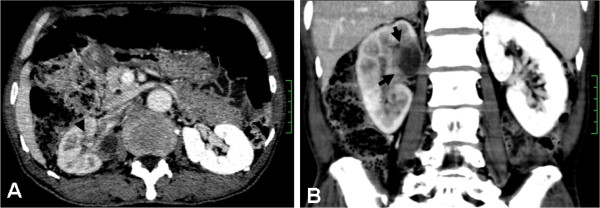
CT images. (A) Axial contrast-enhanced nephrographic-phase CT image obtained at level of renal hilum shows an ill-defined, heterogeneous mass with central cystic change involving the parenchyma of the right kidney, with extension into the renal pelvis and right vein. (B) Reconstructed coronal contrast-enhanced CT image obtained during the excretory phase shows the compression and distortion of the renal pelvis.
